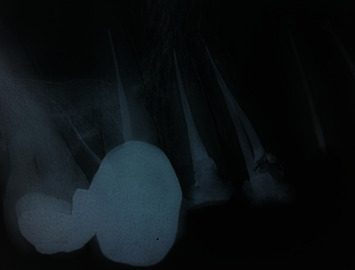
Periapical radiographic view of patient's teeth.
